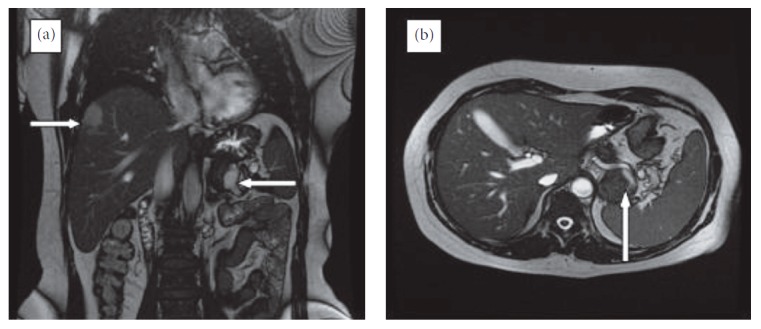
Magnetic resonance imaging of the hepatic hemangioma ((a) upper right arrow) and splenic artery aneurysm.
